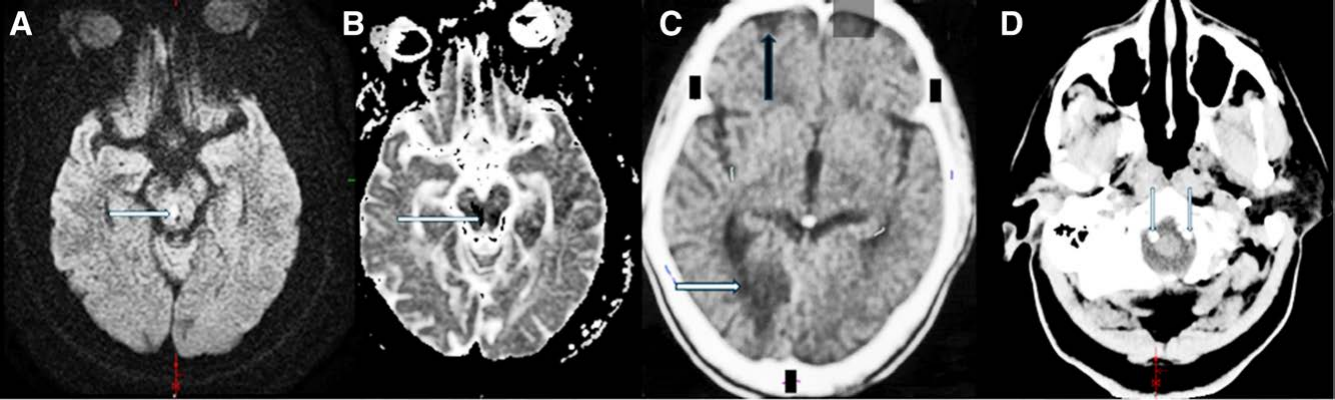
The images (A and B above) show high signal in mid-brain area next to cerebral aqueduct (b1000 A image, long white arrow) and low signal in same area (A–D image, long white arrow), reflecting infarction. CT images (C and D) reveal calcifications on both vertebral arteries (2 white arrows on D image) and sulci widening with infarction on posterior cerebral artery territory (C image black and white, respectively). CT = computed tomography.
